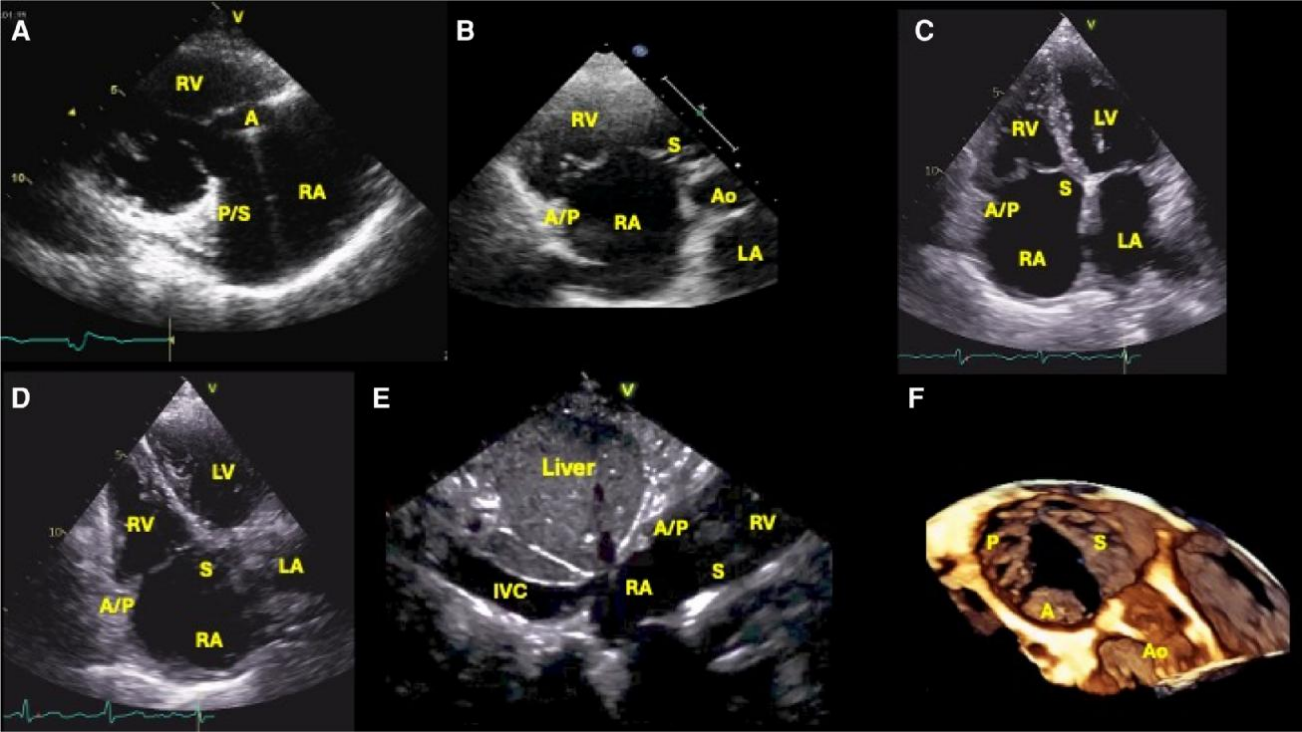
Systematic TTE for the tricuspid valve: essential views. (*A*) Long parasternal axis depicting RV inflow; (*B*) parasternal short-axis view at the level of the great vessels; (*C*) conventional four chamber apical view; (*D*) right side focused four chamber apical view; (*E*) sub-costal long-axis view focused on the right atria and the inferior vena cava; (*F*) 3D en-face view of the TV from the right atria. A, anterior leaflet of the tricuspid valve; Ao, aortic valve; IVC, inferior vena cava; LA, left atrium; LV, left ventricle; P, posterior leaflet of the tricuspid valve; RA, right atrium; RV, right ventricle; S, septal leaflet of the tricuspid valve.
